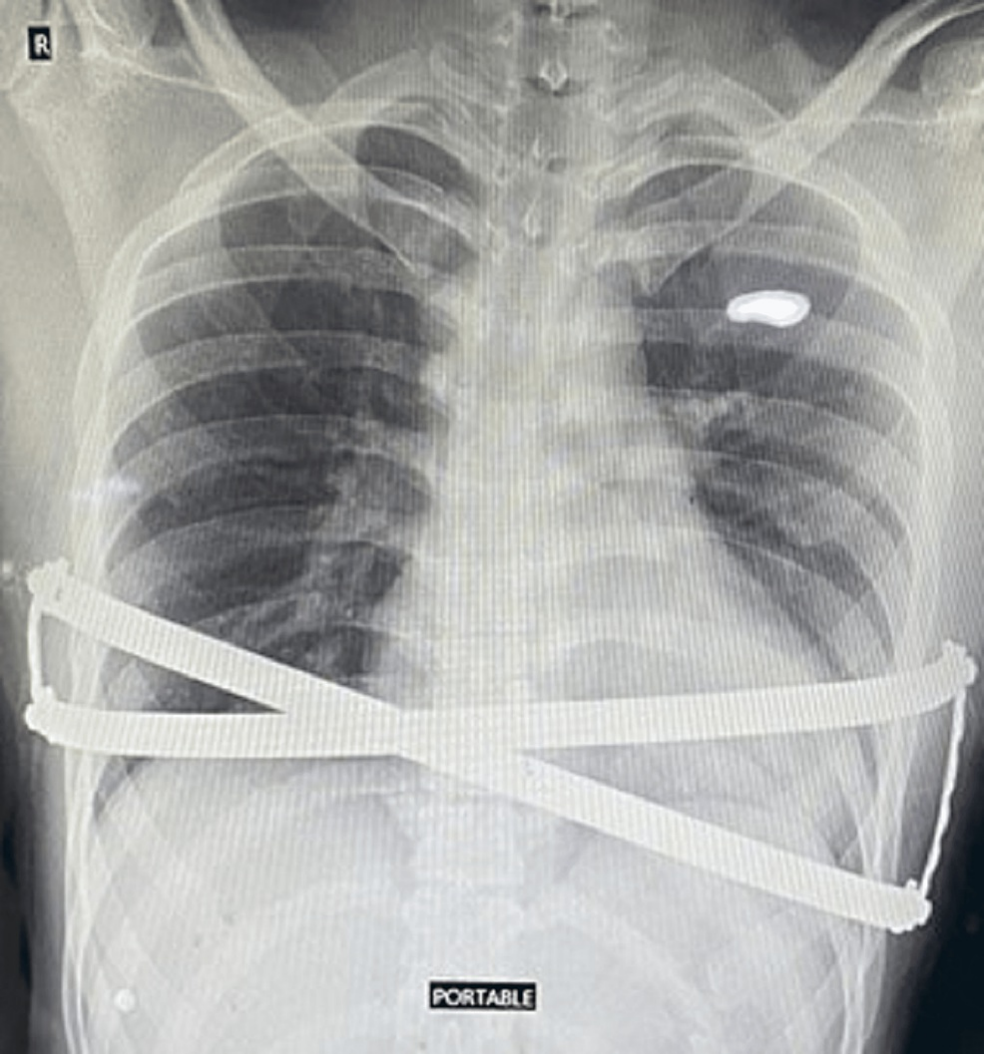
Post-operative X-ray image of the chest X-ray picture of the patient's chest after the Nuss procedure.
